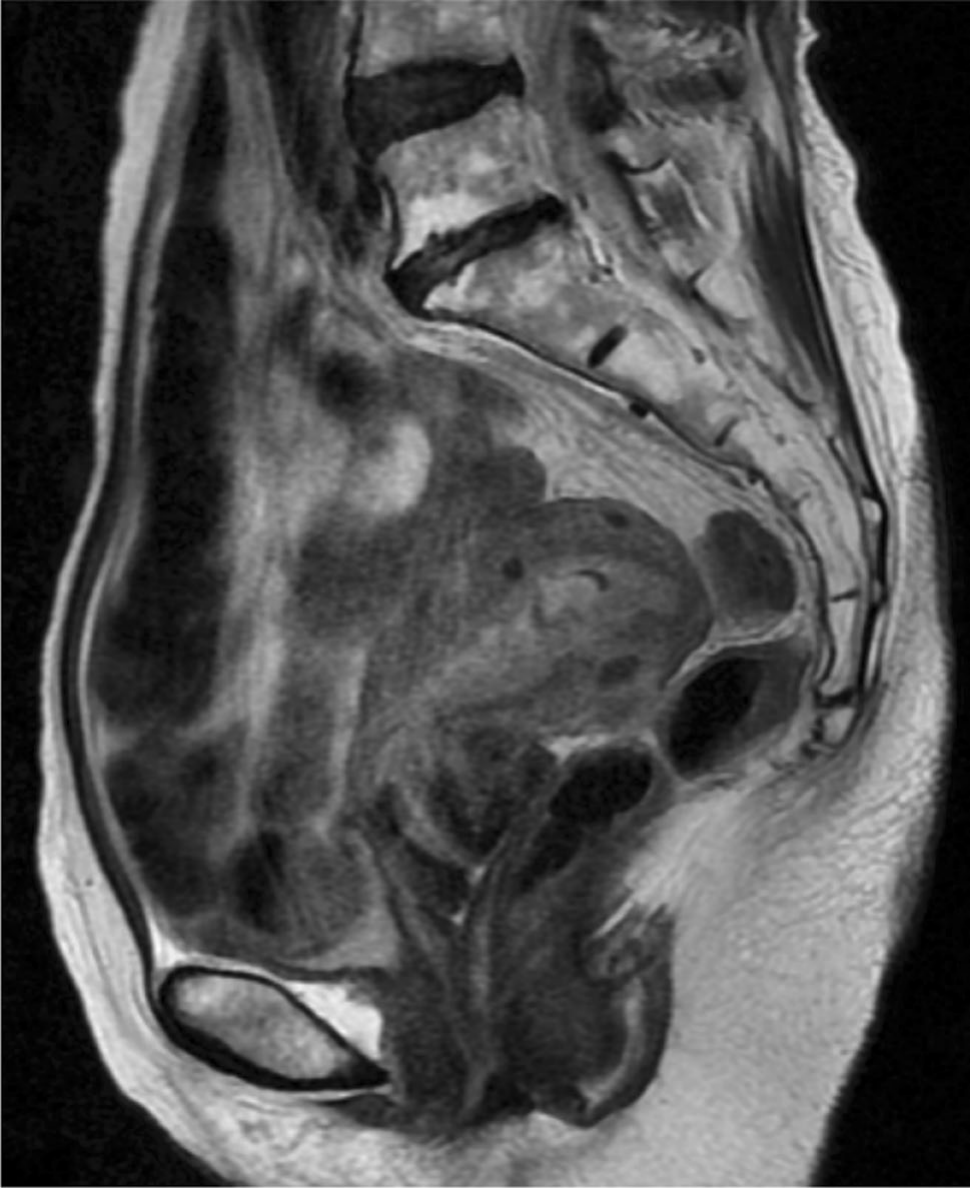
Sagittal T2-weighted MRI findings. The thickened and uneven endometrium lining was observed. *MRI* magnetic resonance imaging
